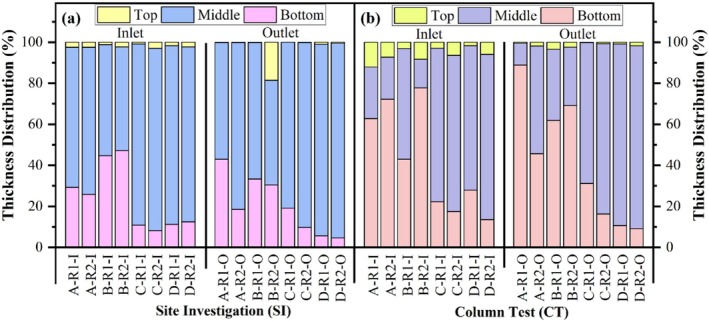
Comparative analysis of (a) site investigation (SI) and (b) laboratory column test (CT) results, showing the average percentage thickness distribution of the top, middle, and bottom layers for Grease Traps A–D. Data represent the mean values for inlet and outlet chamber samples obtained across two sampling rounds (R1 and R2).
